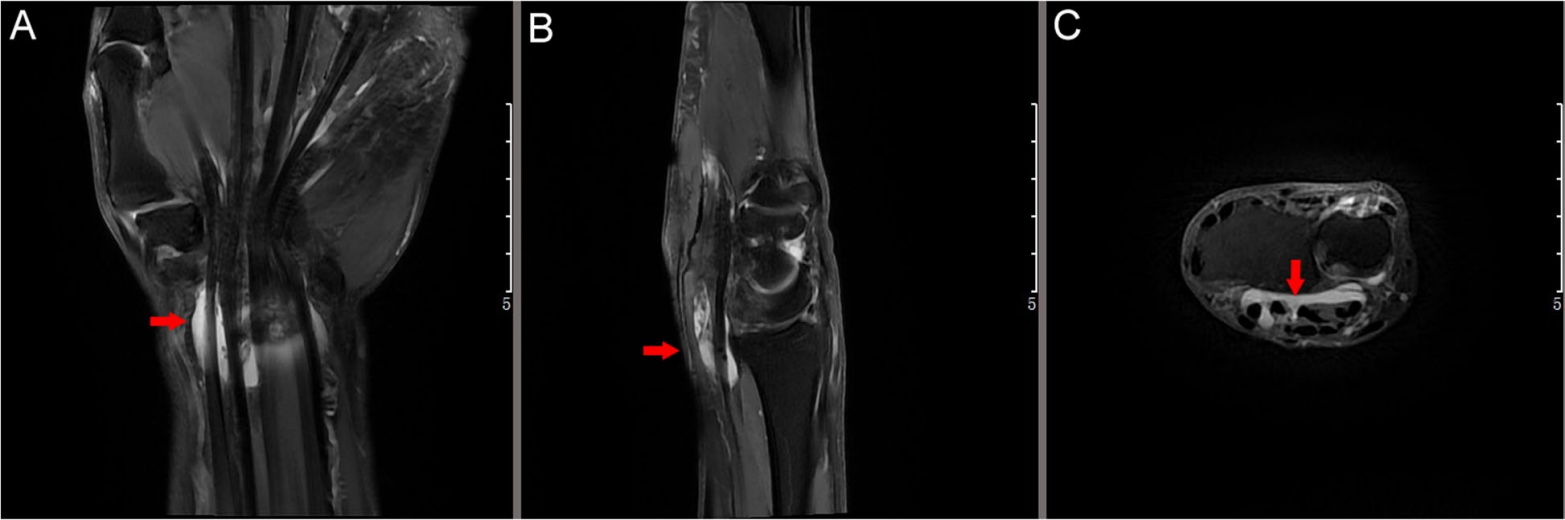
Preoperative magnetic resonance imaging shows a large number of inflammatory synovial hyperplasia in the carpal tunnel, as shown by the red arrow in the image. A Positive view, B Lateral view, C Sagittal position view
